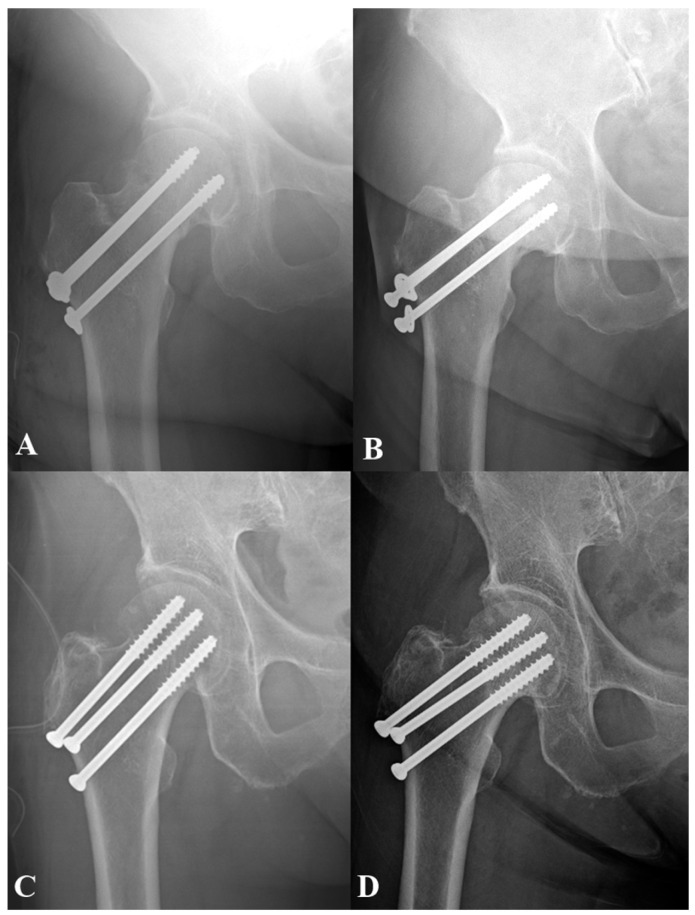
Immediate postoperative X-ray of an 84-year-old woman with a 31B1.2 fracture treated with the conventional short-threaded screw technique (A) and the corresponding twelve-month follow-up X-ray (B), showing significant screw sliding and femoral neck shortening with avascular necrosis of the femoral head. Immediate postoperative X-ray of an 86-year-old woman with a 31B1.2 fracture treated using the long-threaded screw technique (C) and the corresponding twelve-month follow-up X-ray (D), demonstrating no evidence of femoral neck shortening or screw sliding.
